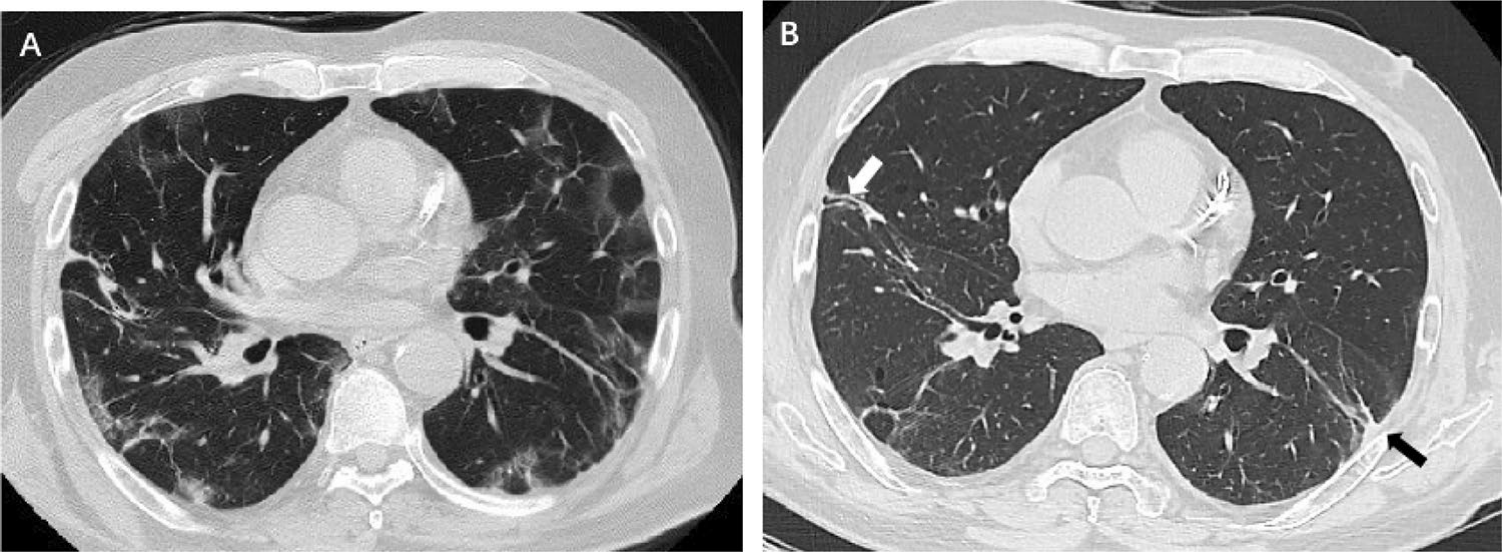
Serial chest CT scans in a 54-year-old man with coronavirus disease 2019 pneumonia. (A) Initial CT scans obtained on day 9 after the onset of symptoms showed multiple ground-glass opacities and interstitial thickening bilaterally. (B)CT scans obtained on day 169 showed traction bronchiectasis (white arrow) and parenchymal bands (black arrow) in the lower lung lobes.
